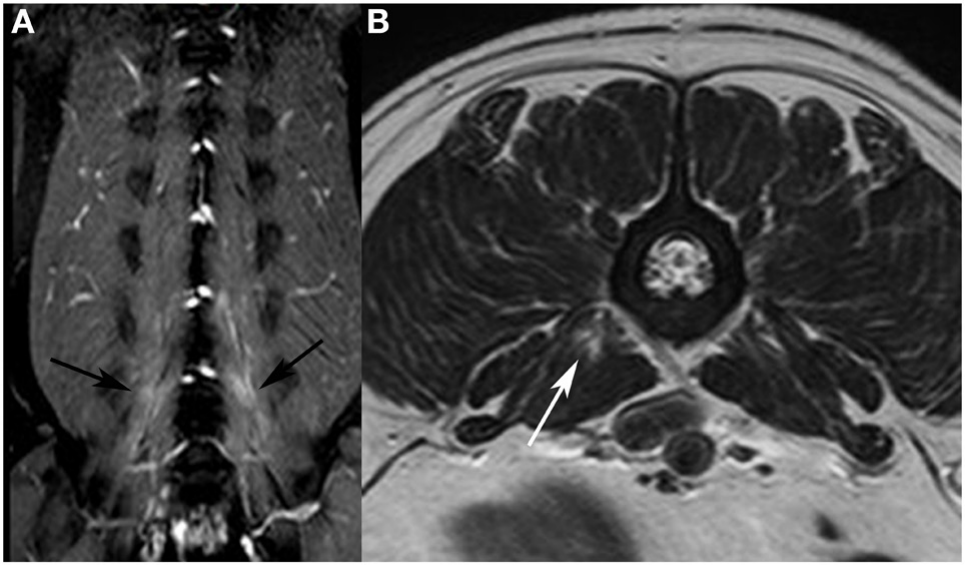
Dorsal T2-weighted (T2W) fat-suppressed image of a French bulldog presented with intervertebral disk extrusion at the level L5/L6. (A) bilateral signal alterations are visible in the hypaxial muscles (black arrows). On the T2W fast spin echo transverse image (B), only a focal area of increased signal intensity is visible in the *M. psoas major* on the right side (white arrow).
